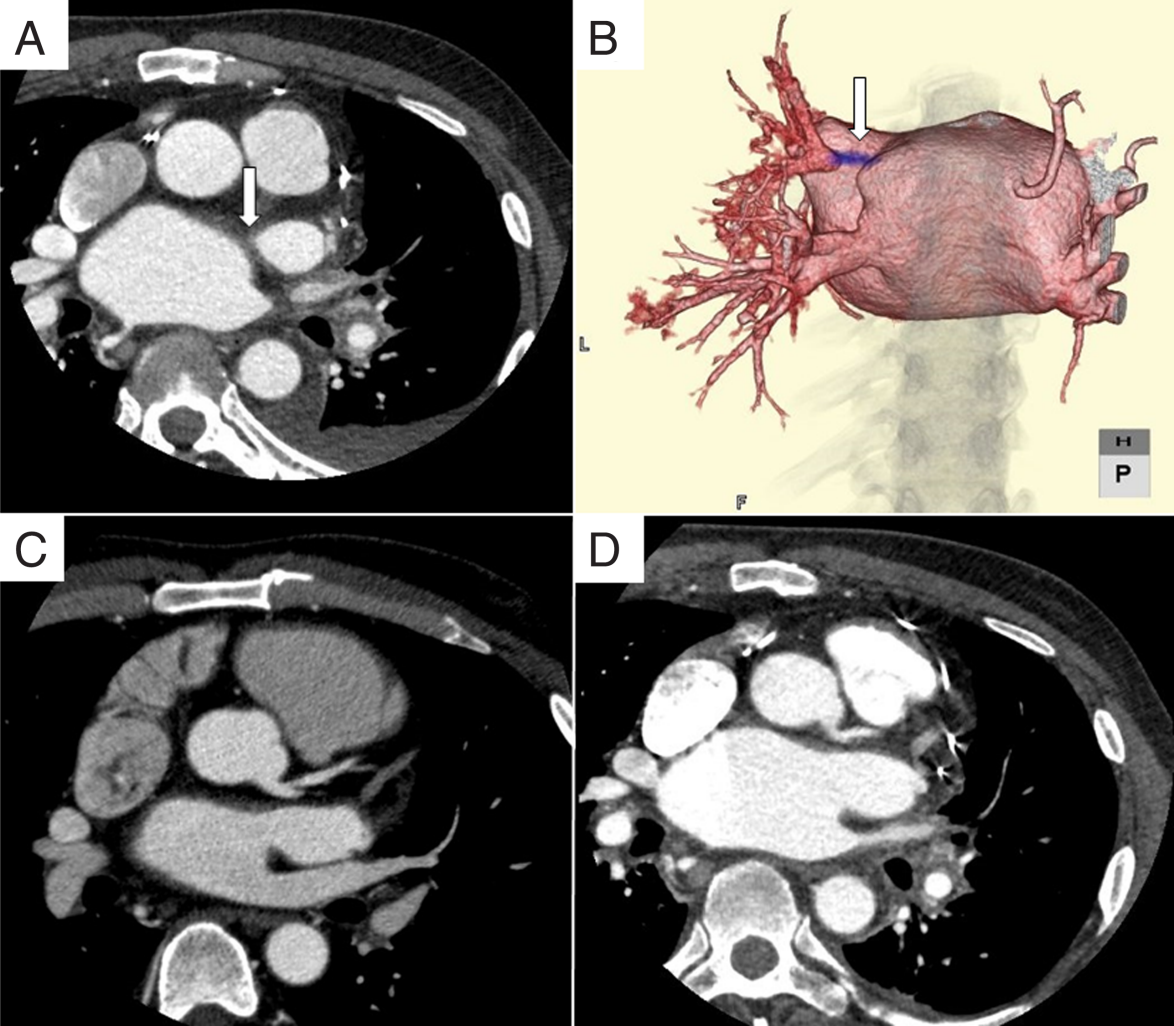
(A) Contrast‐enhanced computed tomography (CT) showing left superior pulmonary vein stenosis (PVS) (arrow). (B) A reconstructed posterior view contrast‐enhanced CT image of the left superior PVS (arrow). (C) Contrast‐enhanced CT showing that the left superior pulmonary vein is intact before the maze procedure. (D) Contrast‐enhanced CT showing the improvement in left superior PVS after catheter‐balloon angioplasty.
